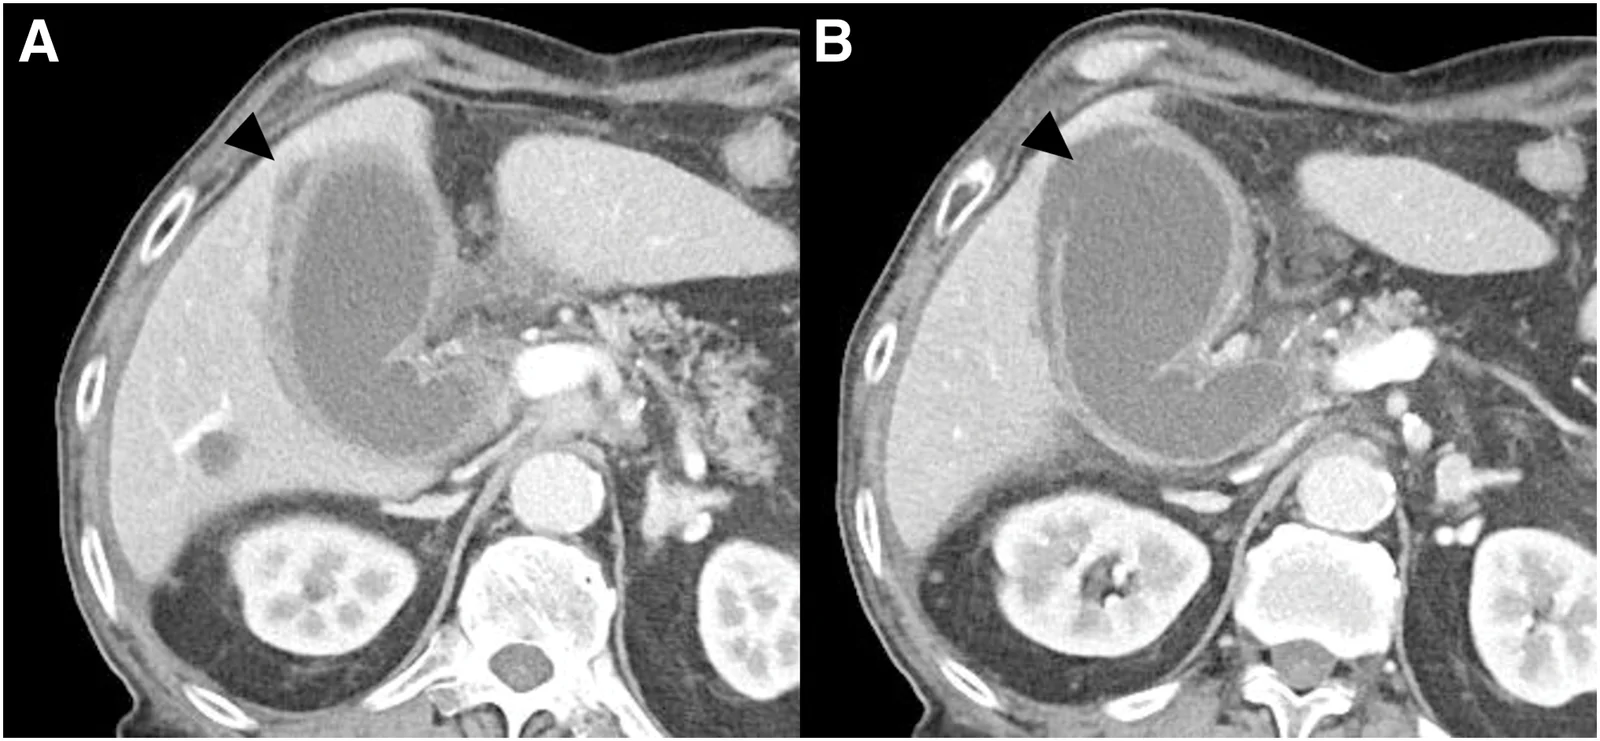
Contrast-enhanced CT findings. (A) Pericholecystic fluid collection (arrowhead) with irregular thickening of the gallbladder wall. (B) Focal non-enhancement of the gallbladder wall (arrowhead). The findings were suggestive of gangrenous acute cholecystitis.
